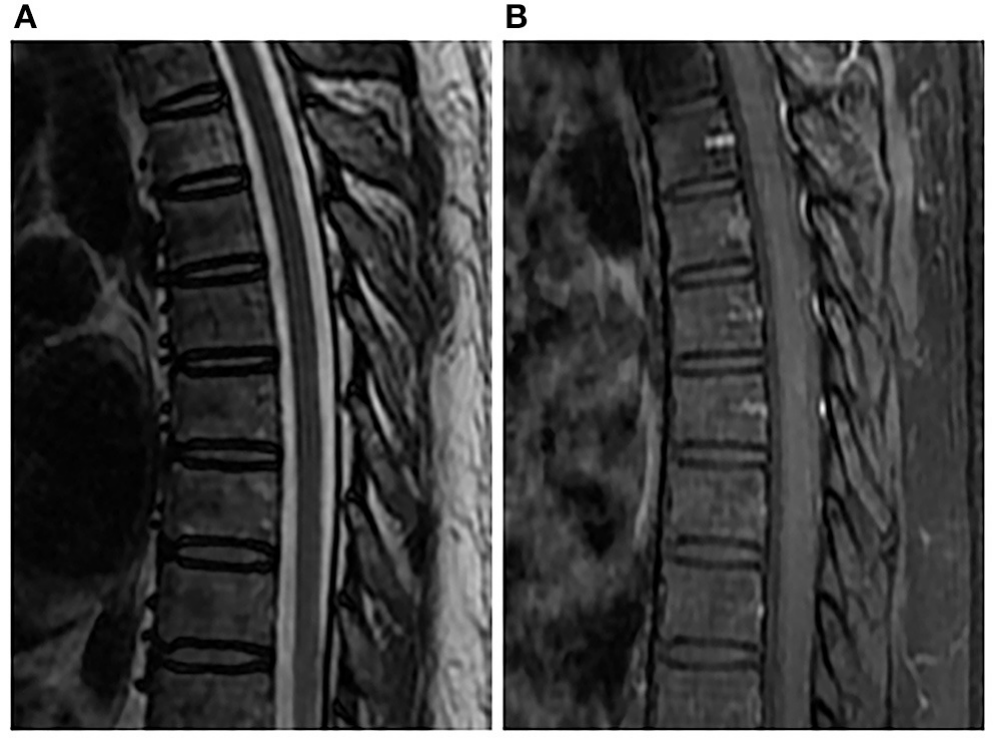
Spinal MRI performed 6 months after penicillin therapy. Sagittal T2-weighted image (A) and enhanced T1-weighted image (B) showed normal spinal cord.
